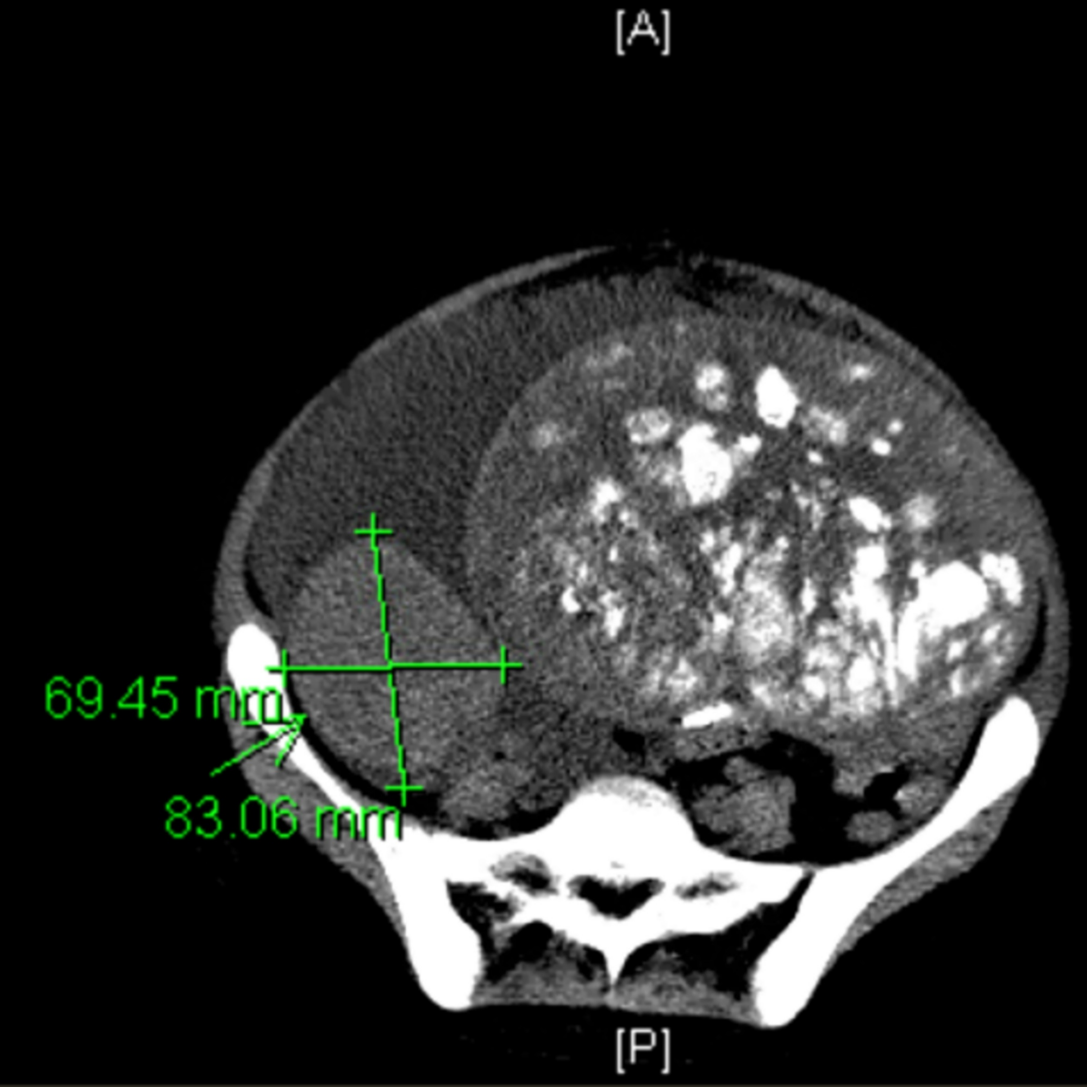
CT scan of the abdomen showing a right-sided pelvic mass
